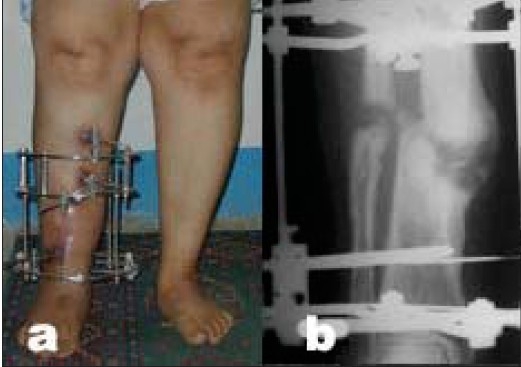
(a,b) Clinical photograph and radiograph (anteroposterior view) result after complete deformity correction in consolidation phase of the distraction gap while weight bearing is allowed.
